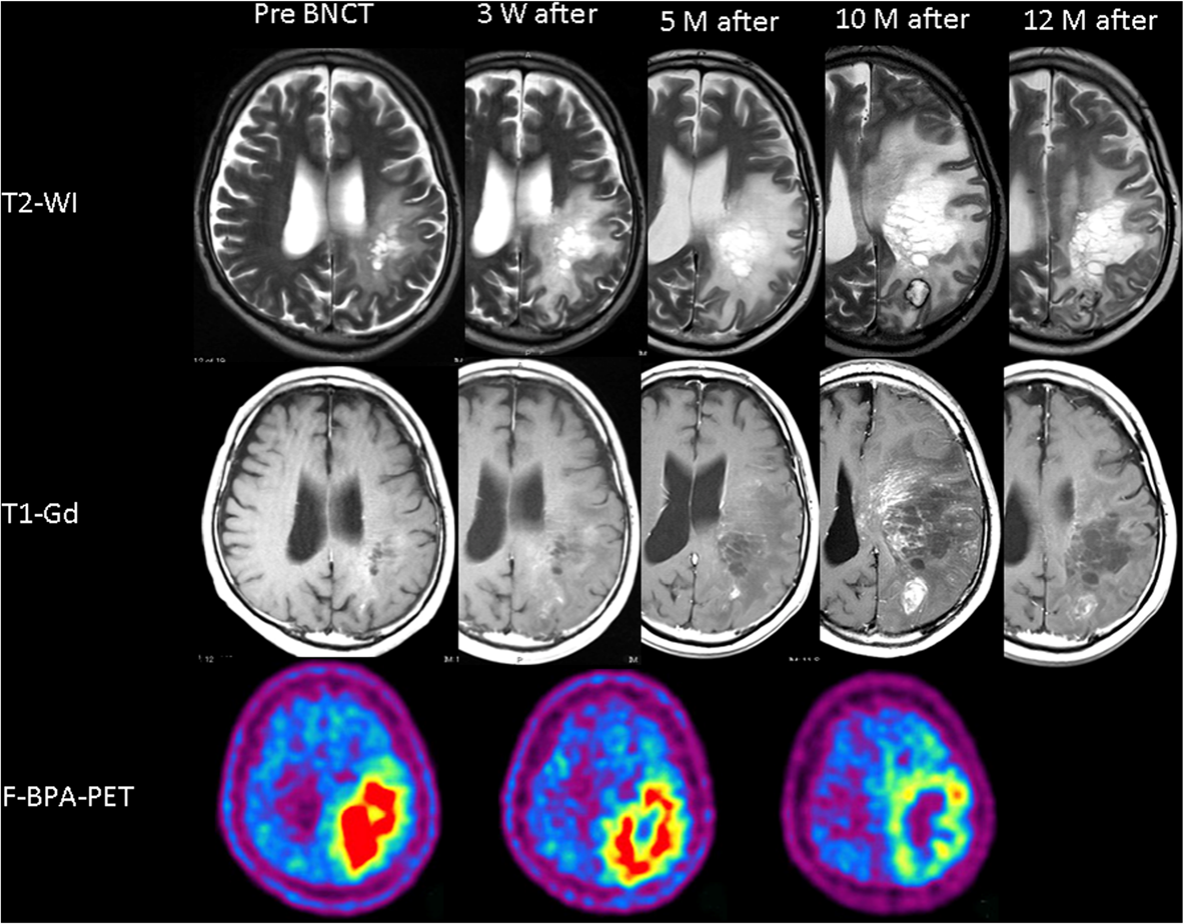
Sequential change of T2-weighted MRI (upper column), Gd-enhanced T1-weighted MRI (middle column) and F-BPA-PET (lower column) of Case 1, a 44-year-old male. The timing of the MRIs is depicted above the MRIs. F-BPA-PET images were taken just before the BNCT and at 1 month and 10 months after the BNCT. These PET images show the gradual decrease of the tracer uptake as a promising effect of the BNCT. BV was started 10 months after the BNCT, and the MRI showed marked improvement of both perifocal edema and contrast enhancements by BV treatment.
